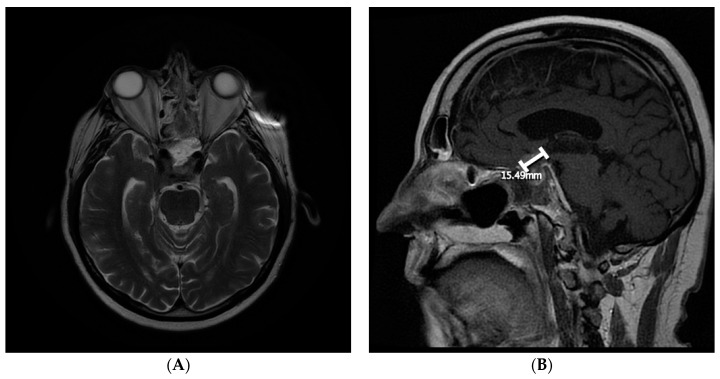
Follow-up postsurgical magnetic resonance imaging of the brain (September 2018) revealing subtotal surgical excision of the sellar metastatic lesion. (A) Axial view; (B) sagittal view.
